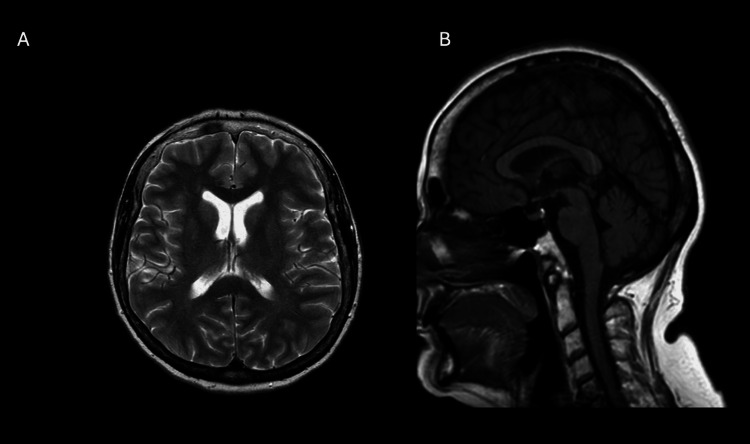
Repeat MRI head demonstrating no abnormalities (A) Axial T2-weighted view and (B) T1-weighted sagittal view.
